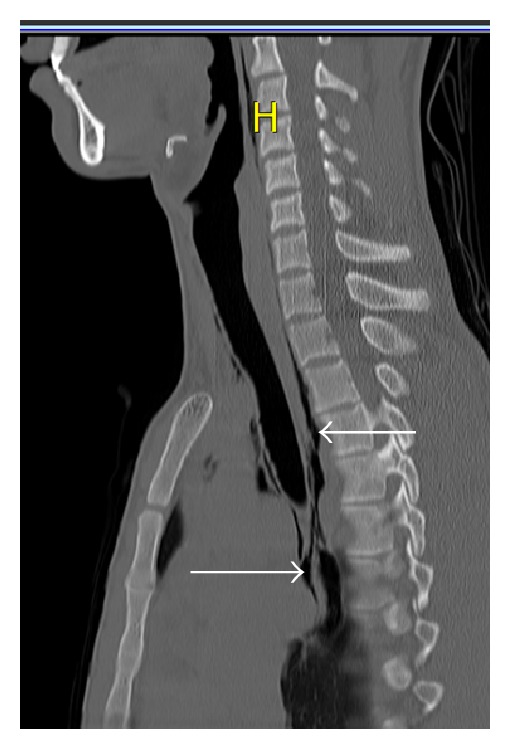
Neck and upper mediastinal CT scan, sagittal section; free air is present around trachea and in mediastinum (arrows).
